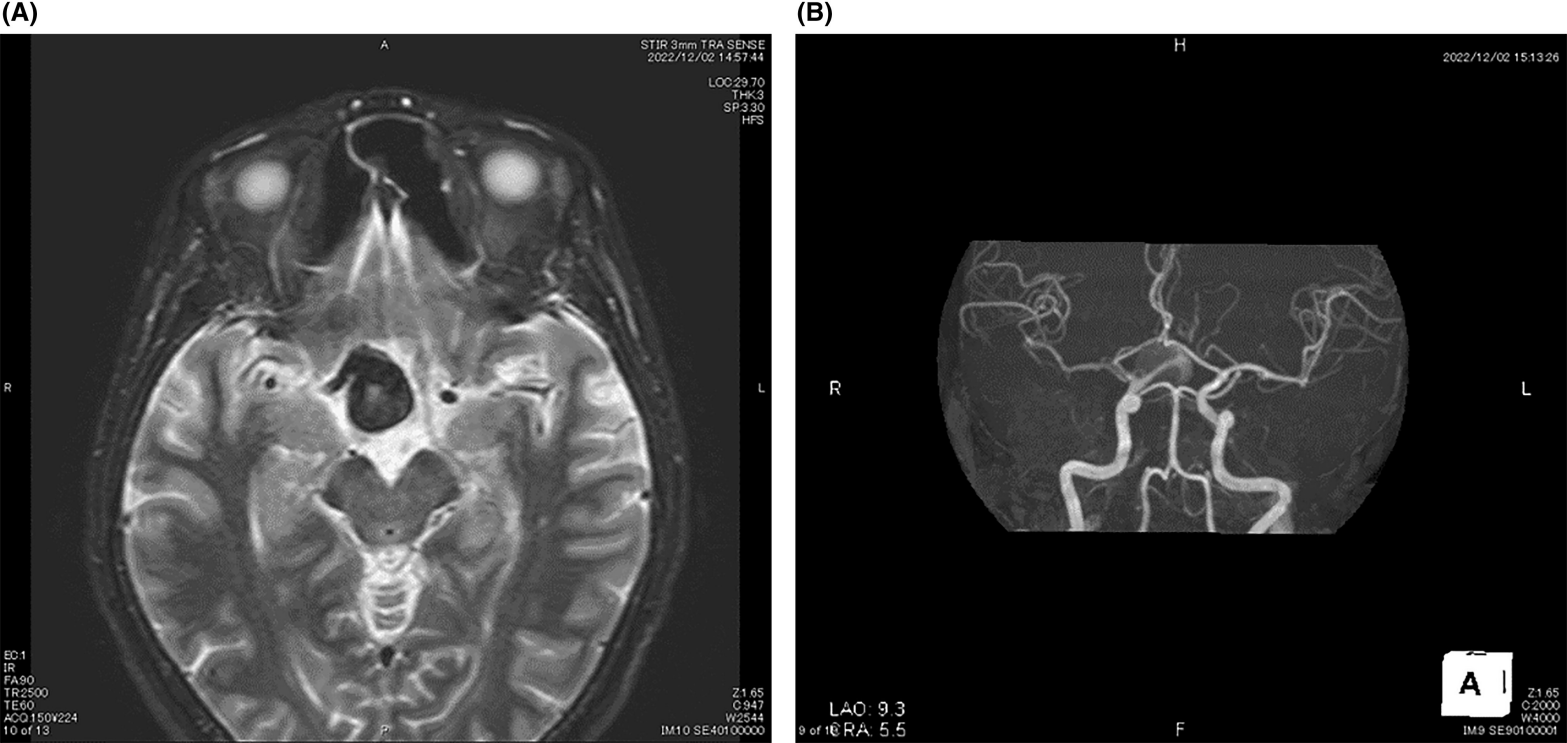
Brain magnetic resonance imaging findings. (A) Axial image showing a large unruptured aneurysm sized 18 × 17 mm extending from the right internal carotid artery. (B) Magnetic resonance angiography image showing a large unruptured aneurysm originating from the right internal carotid artery.
